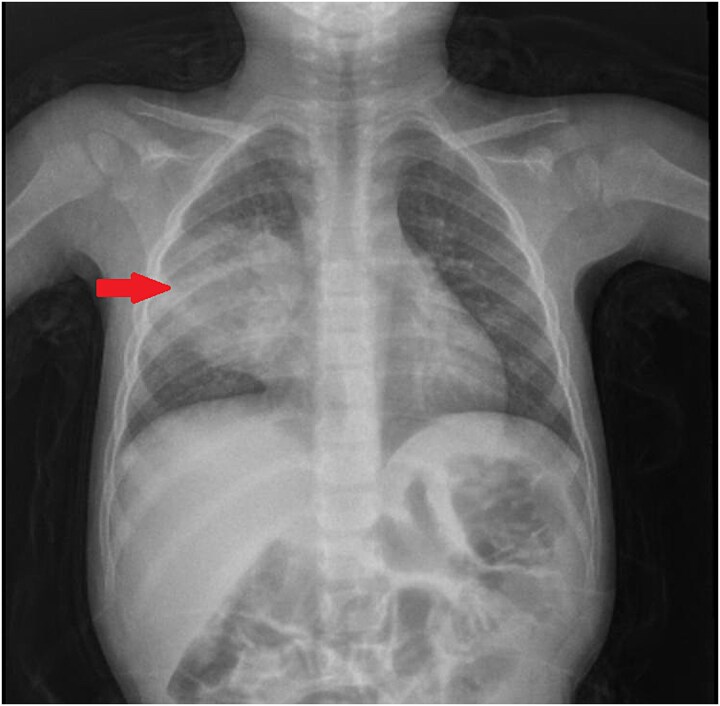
Chest radiograph demonstrating right upper and middle lobe consolidation.
